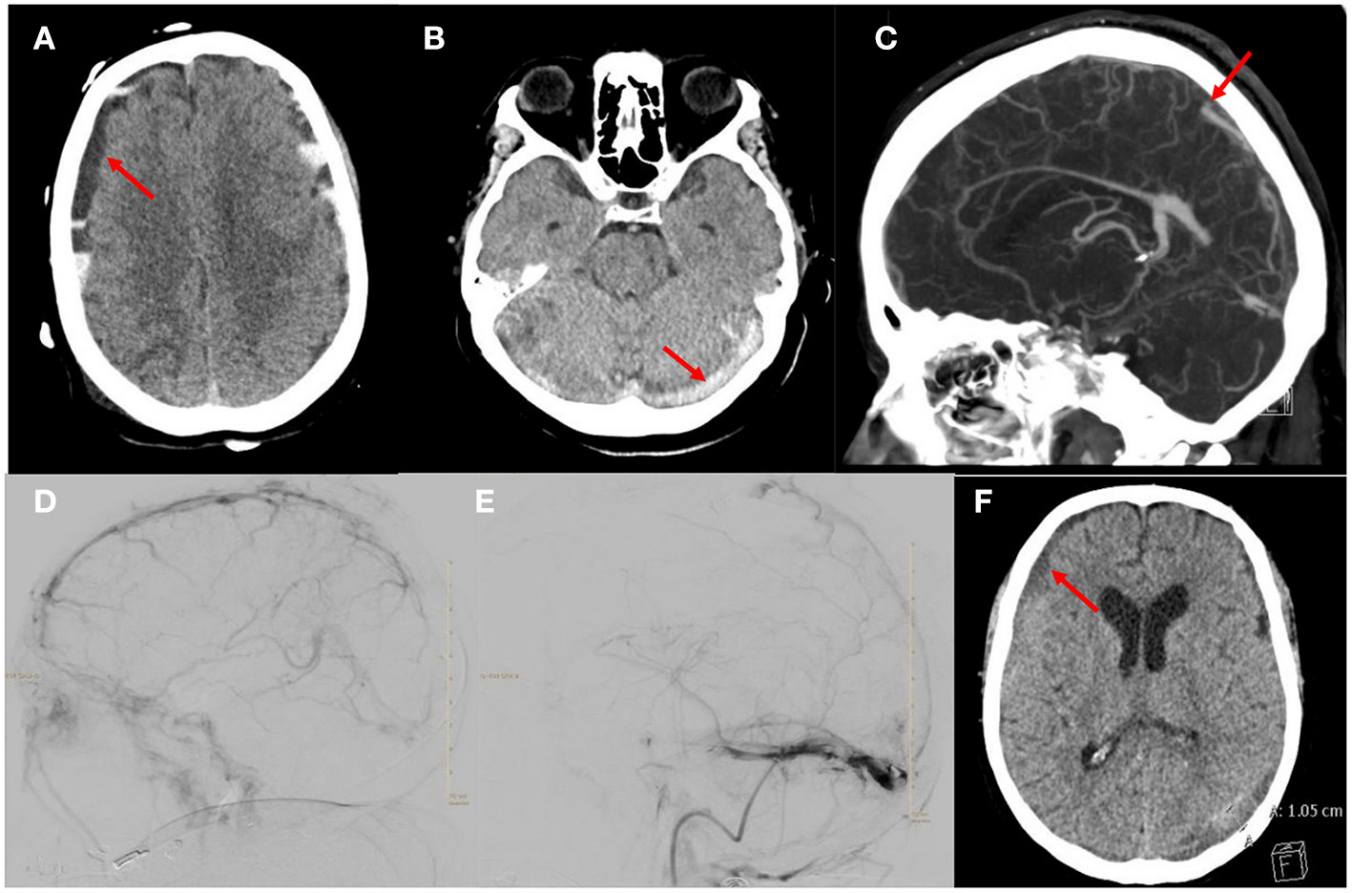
Images from the patient who received mechanical thrombectomy and anticoagulation for aSDH with CVST. (A) Axial head CT demonstrating the mixed density acute subdural hematoma. (B) Axial head CT demonstrating hyperdensity in the torcula and transverse sinuses suggestive of acute thrombosis. (C) Sagittal CTV demonstrating occlusion of the SSS, straight sinus and torcula along with generalized venous congestion. (D) Right ICA injection, lateral projection of late venous phase demonstrating thrombus in the SSS and partial occlusion of the straight sinus and occlusion of the transverse sinuses. (E) Venogram of right sigmoid sinus, lateral projection after thrombectomy and tPA infusion showing improvement in drainage of the SSS and transverse sinus. Note there is a persistent non-occlusive thrombus of the transverse sinus. (F) Axial head CT demonstrating decreased size of the subdural hematoma.
